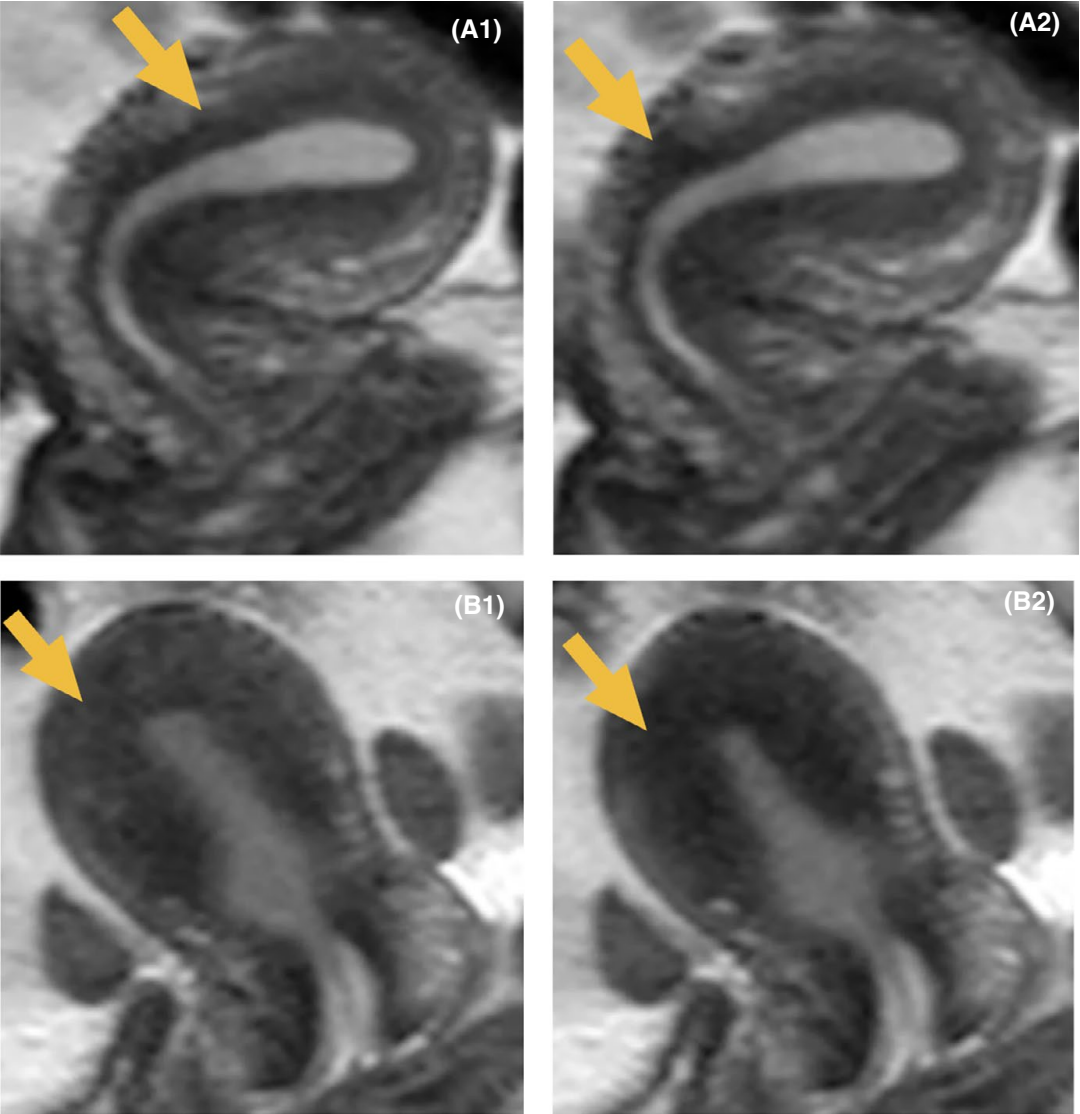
Magnetic resonance image of uterine contraction. The arrow shows the contraction area. The contraction site is detected as a low‐intensity area. Shifting of the arrow (from a‐1 to a‐2) represents continuous movement of the contraction area in the uterine myometrial layer. In b‐1 and b‐2, three‐fourths of the inner side of the uterine myometrium contracted
